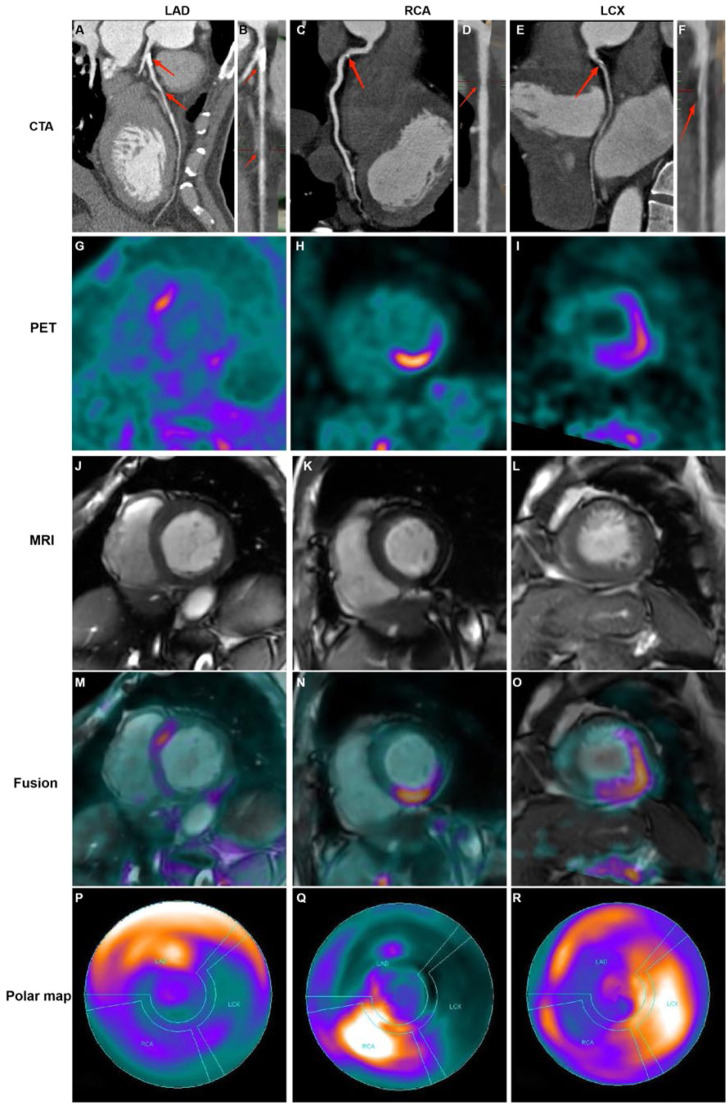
Representative images in participants with coronary stenosis. Curved reconstruction map (A, C, E) and probe map (B, D, F) obtained from Computed Tomography Angiography (CTA) showed the vascular stenosis (indicated by red arrow). ^18^F-FAPI PET images (G, H, I), MRI images (J, K, L), and PET/MR fusion image (M, N, O) in short-axis cine showed that FAPI was up-taken in a certain slicer. The distribution of FAPI-uptake in the left ventricle was presented with a polar map (P, Q, R). The first column showed images in a 42-year-old man with 50% stenosis in Left Anterior Descending coronary artery (LAD) and 30% stenosis in Right Coronary Artery (RCA). In the short-axis cardiac PET/MR fusion images, the uptake of FAPI in the septum was higher (M), and in the polar map of PET images, the anterior and septal wall had higher uptake (P). The second column showed images in a 61-year-old woman with 40% stenosis in RCA. FAPI-uptake increased in the inferoseptal and inferior wall in the short-axis cardiac PET/MR fusion images and the polar map of PET images (N and Q). The third column showed images in a 67-year-old man with 50% stenosis in Left Circumflex Artery (LCX), 30% stenosis in LAD and 30% stenosis in RCA. The FAPI uptake was significantly increase in the lateral wall, and slightly increase in the anterior, septal and inferior wall in the short-axis cardiac PET/MR fusion images and the polar map of PET images (O and R).
